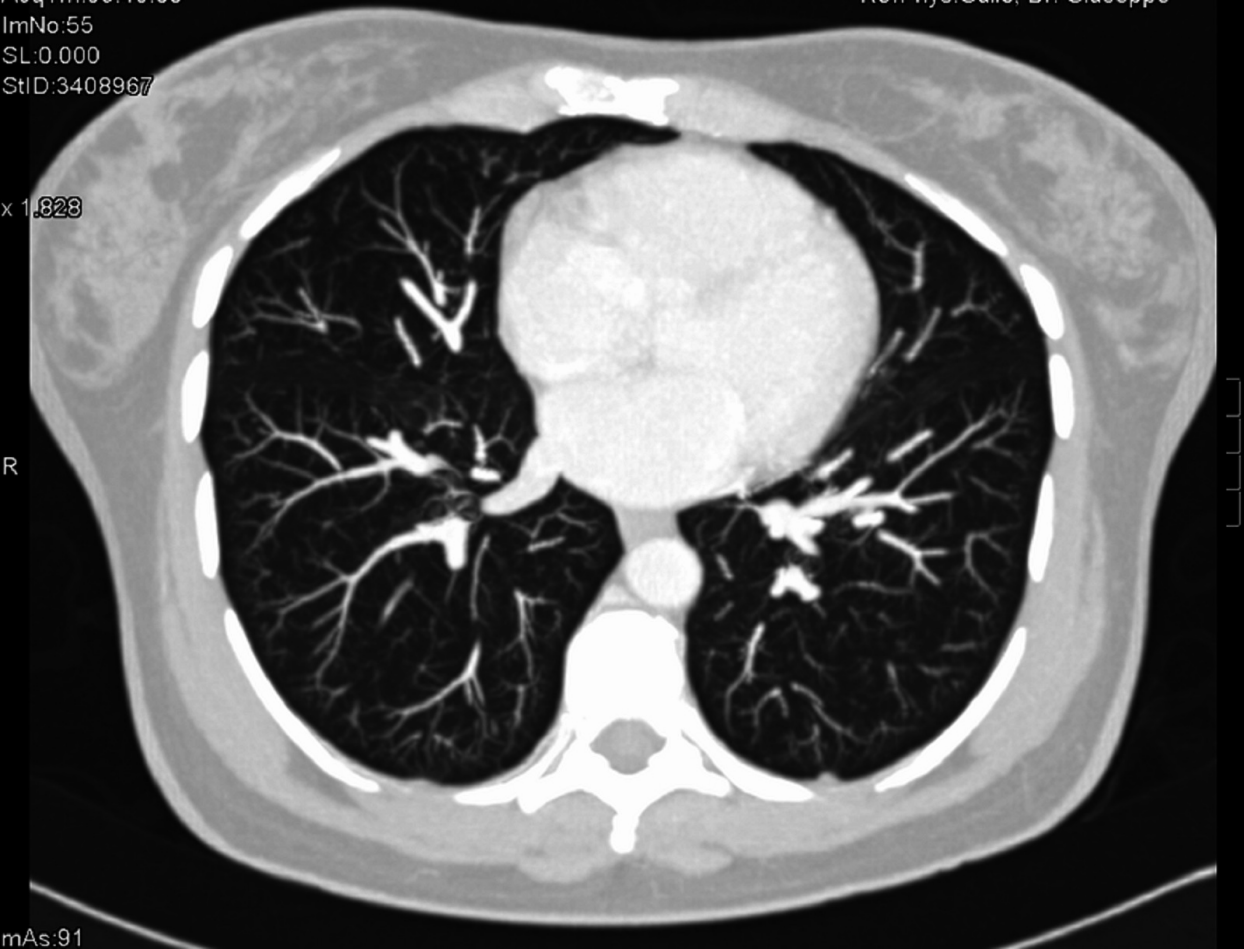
Patient 2: Computed tomography (CT) imaging demonstrates resolution of lung nodules post treatment
